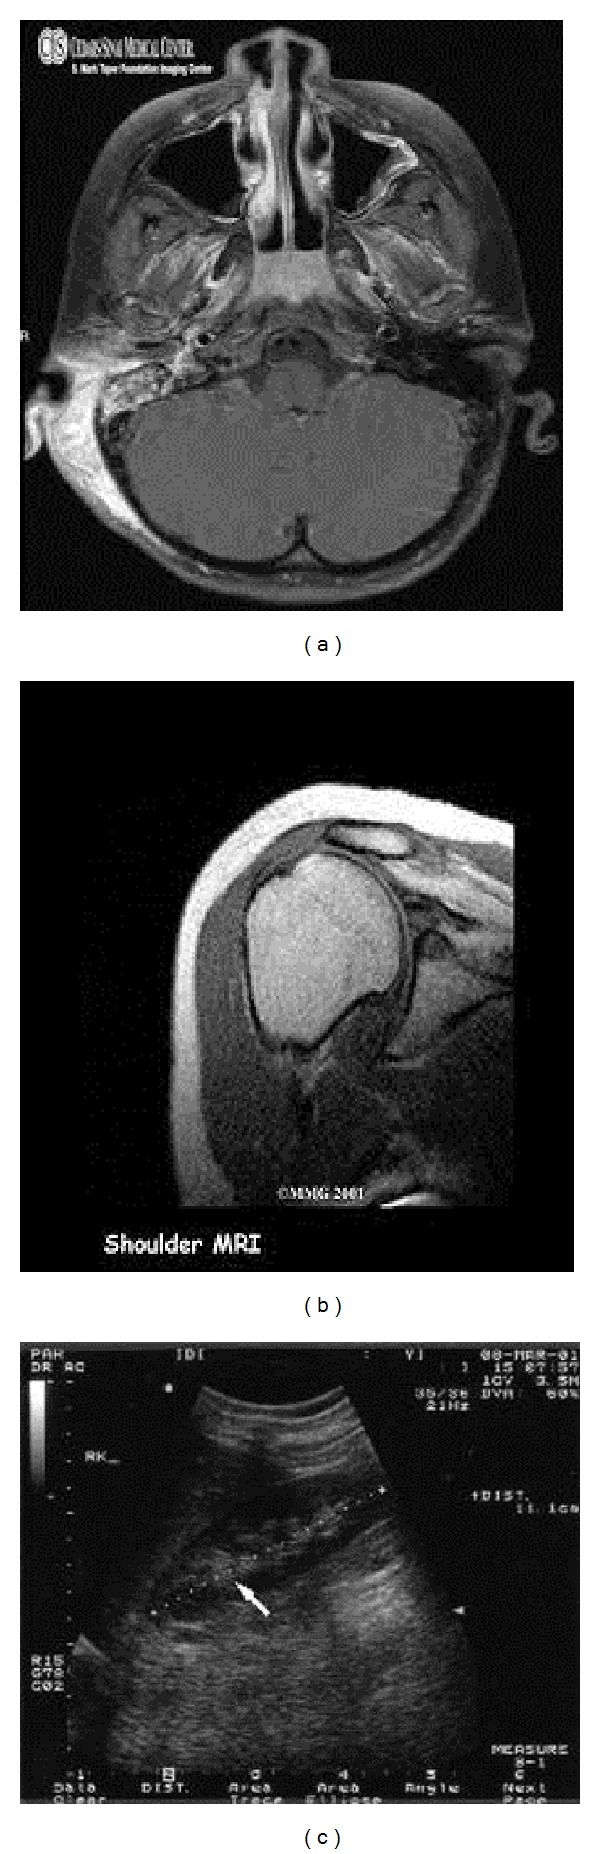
Recovered medical images (from left to right: CT scan, MRI scan, and ultrasound).
